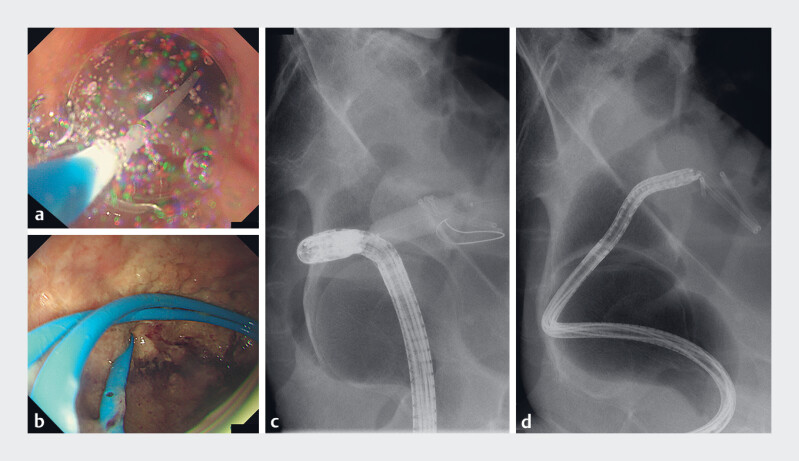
Retrieval of the migrated self-made plastic stent using an endoscopic ultrasound (EUS)-guided pelvic abscess drainage technique.
